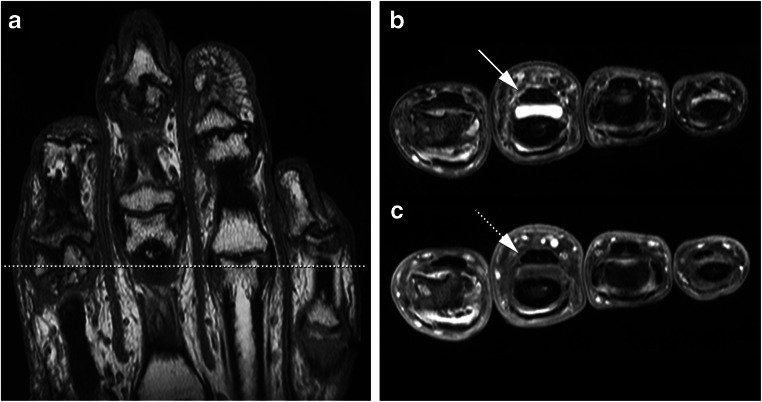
a Coronal T1-weighted image without contrast enhancement of the right hand, indicating the location of the axial section (b and c). b and c Axial non-contrast-enhanced T2-weighed (T2) (b) and axial Gd-chelate contrast-enhanced T1-weighted (T1-ce) (c) images showing the proximal part of the third proximal interphalangeal joint, with effusion on the T2 image seen as white water signal (b, arrow), with only little enhanced synovial thickening seen as contrast enhancement on the T1-ce image (c, dashed arrow) which is not enough for a score “1”
